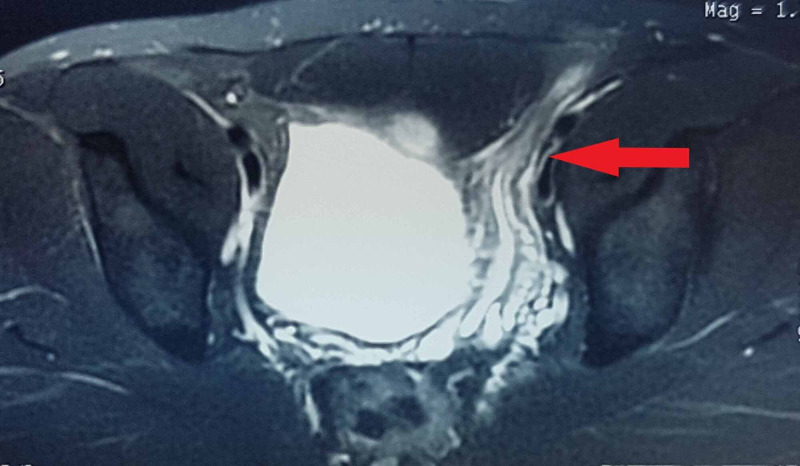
MRI T2-weighted fat saturation images showing a hyperintense tubular structure in pelvis representing thickened left spermatic cord.
